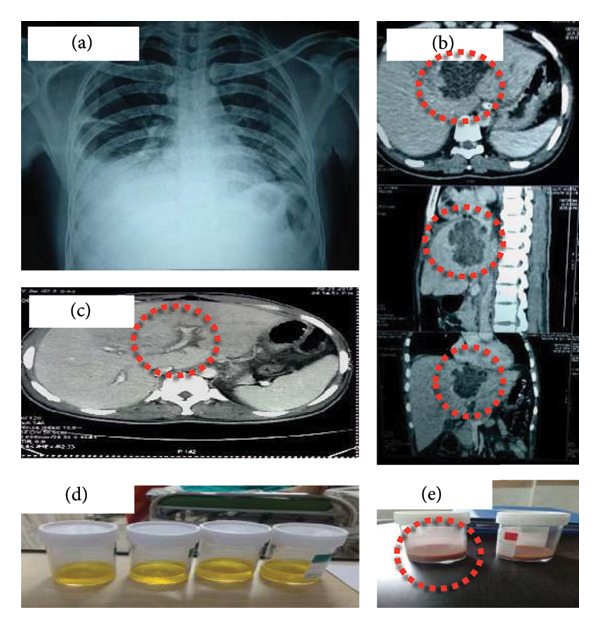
(a) Chest radiograph (posteroanterior view) showing a moderate right‐sided pleural effusion, likely reactive or due to *trans*‐diaphragmatic extension from the adjacent hepatic abscess. (b) Contrast‐enhanced CT of the abdomen demonstrating a peripherally enhancing, centrally hypodense lesion measuring 7.2 × 5.3 × 7.5 cm in the left hepatic lobe, consistent with an amoebic liver abscess. (c) Contrast‐enhanced CT (axial view) showing hypodense filling defects in the middle and left hepatic veins and the suprahepatic segment of the inferior vena cava, indicating hepatic venous thrombosis compatible with Budd–Chiari syndrome. (d) Diagnostic paracentesis revealing straw‐colored ascitic fluid, later confirmed to be exudative on biochemical analysis (elevated protein and LDH). (e) Ultrasound‐guided percutaneous drainage of the liver abscess demonstrating evacuation of thick reddish‐brown pus with the characteristic “anchovy‐sauce” appearance typical of amoebic infection.
